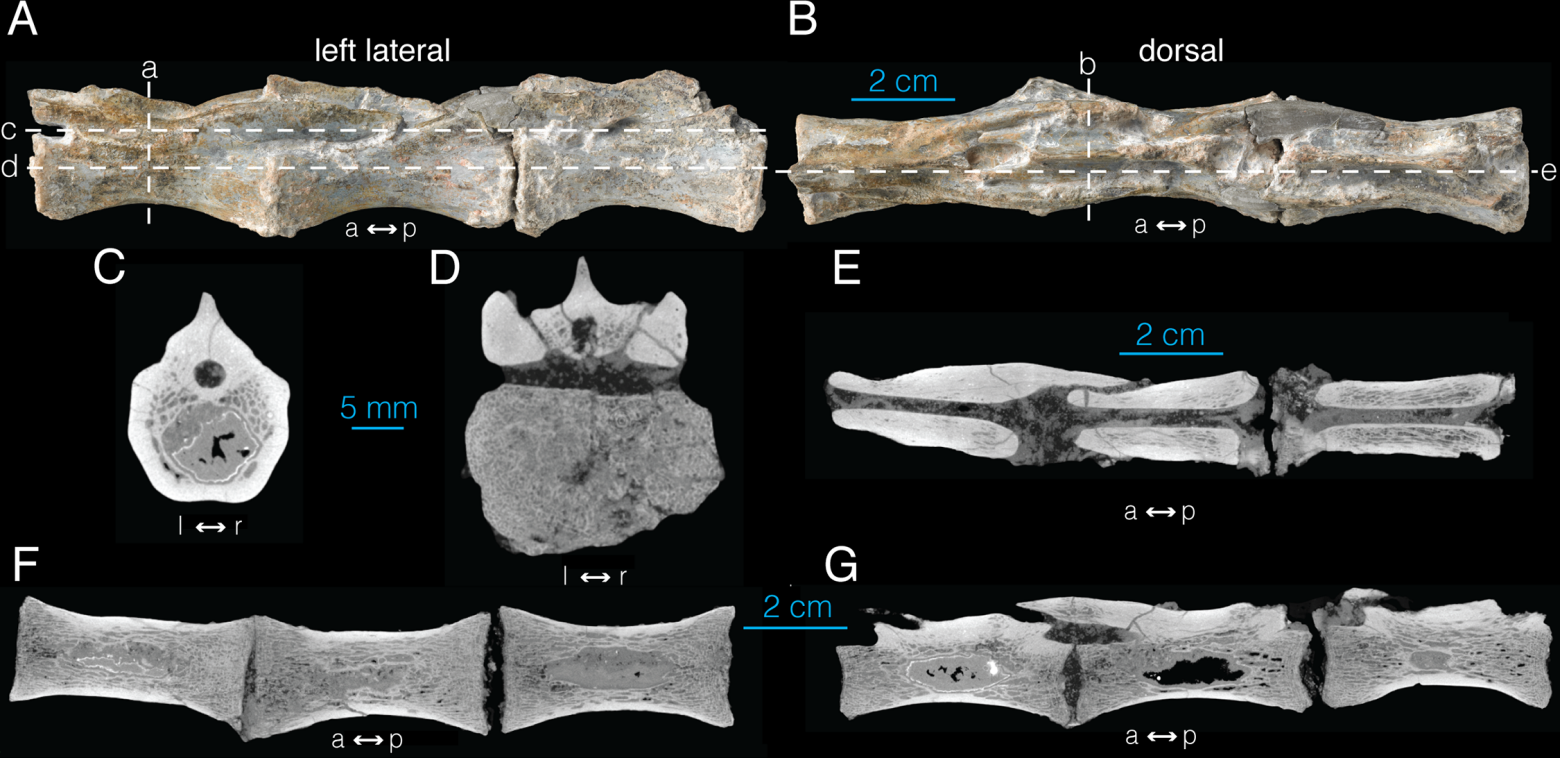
Distal caudal vertebrae of *Archaeornithomimus* (AMNH FARB 21794) and associated CT images. A, left lateral view; B, dorsal view; C, D, select transverse sections; E, frontal plane section; F, G, select sagittal sections. Dashed lines and associated letters indicate location and letter designation of CT image slices.
